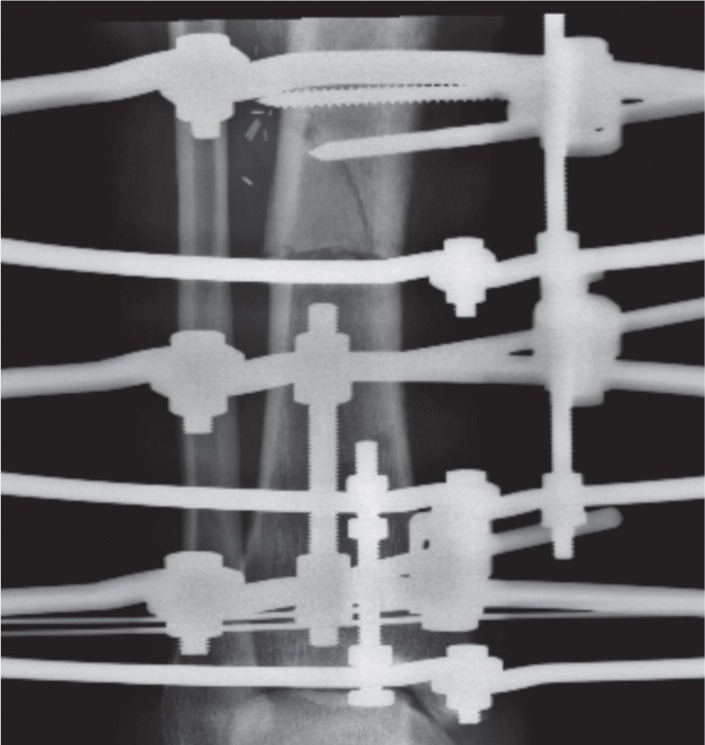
Radiograph showing Type VI corticotomy showing fracture extension into proximal half pin fixation.
